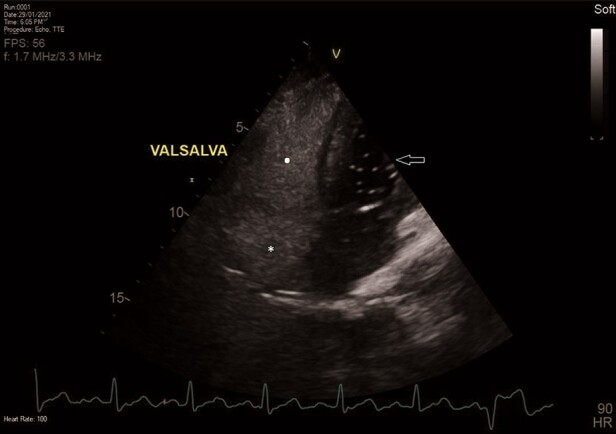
Subsequent transthoracic echocardiography. Two-dimensional apical two-chamber view, after administration of agitated normal saline and Valsalva manoeuvre. The right atrium (asterisk) and the right ventricle (white dot) are fully opacified. However, only a small number of bubbles appeared in the left atrium and left ventricle (arrow). This was indicative of a patent foramen ovale (PFO).
